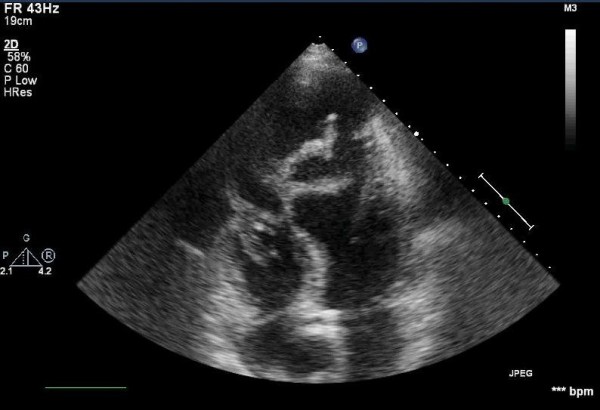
Transthoracic echo showing the peculiar looking mitral valve.
